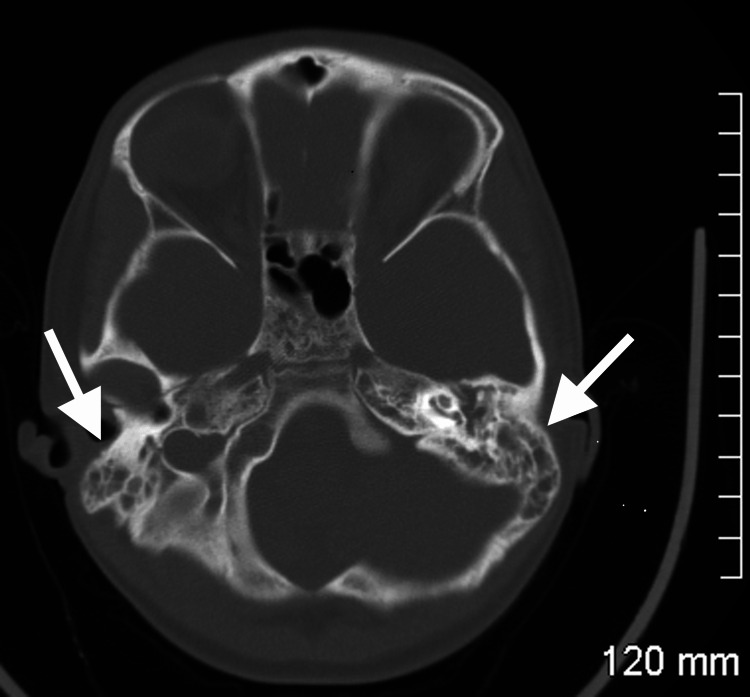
CT scan with visualization of bilateral otomastoiditis. CT: Computerized tomography
